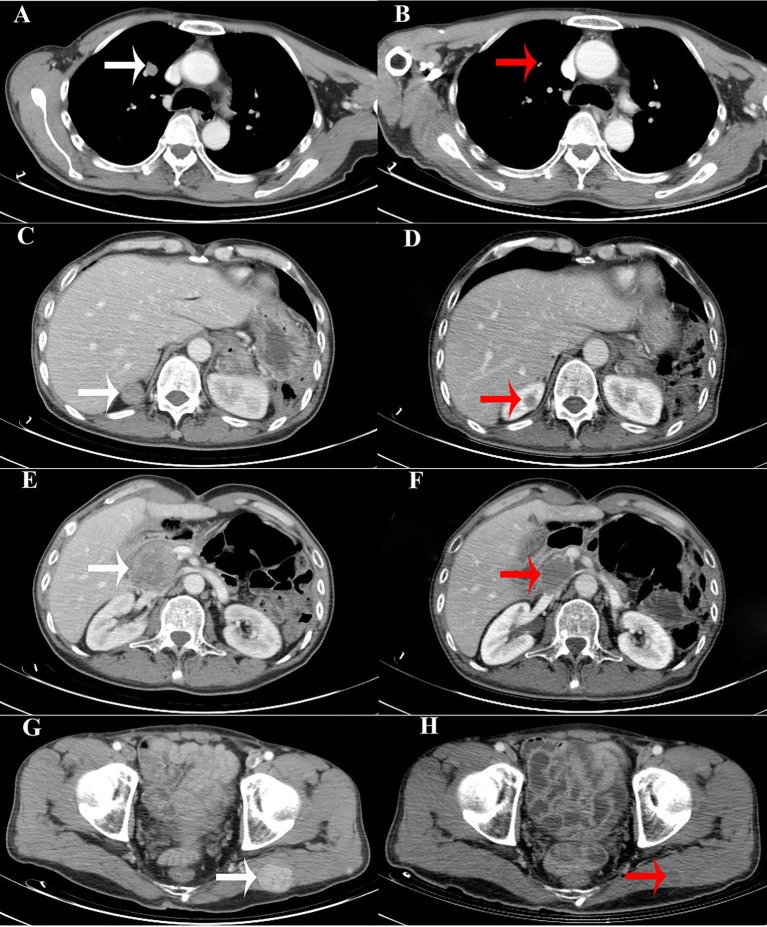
Computed tomography (CT) images showing that the size of the metastatic lesions had decreased. (A,C,E,G). CT images of the metastatic lesions (white arrows) in the right pulmonary, right kidney, pancreatic head, and left gluteus maximus, respectively, before chemotherapy (lenvatinib). (B,D,F,H). CT images of the metastatic lesions (red arrows) after chemotherapy (lenvatinib).
